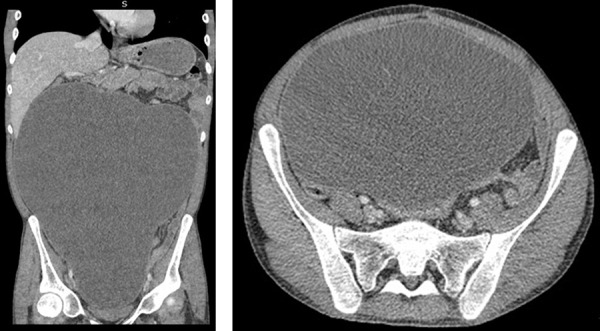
Abdominal computed tomography scan showing a giant encapsulated cystic mass occupying the entire peritoneal cavity and compressing adjacent organs.
